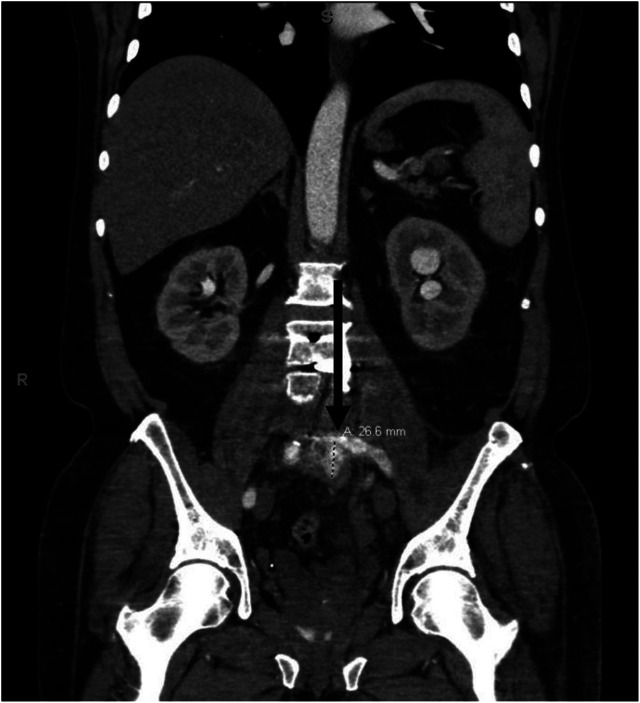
Coronal computed tomography slide with marker indicating 26.6-millimeter left common femoral artery mycotic pseudoaneurysm.
